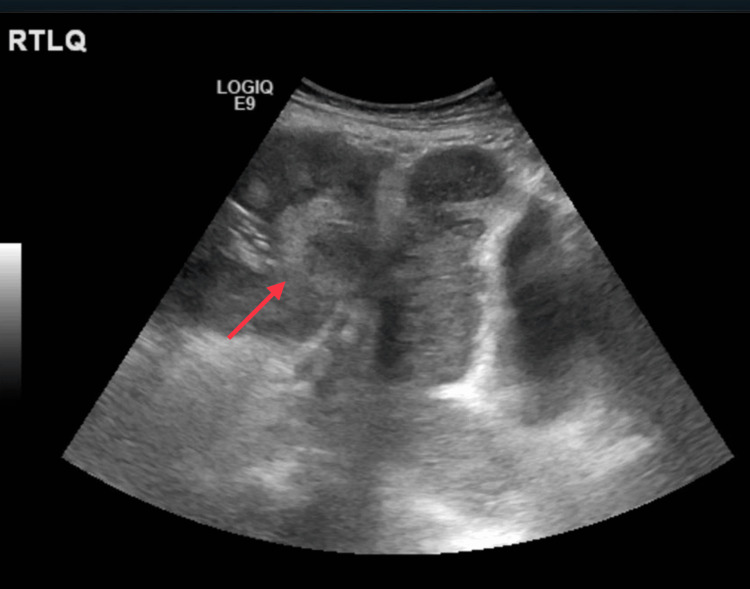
Abdominal ultrasound showing the mass in the right upper quadrant (red arrow)
